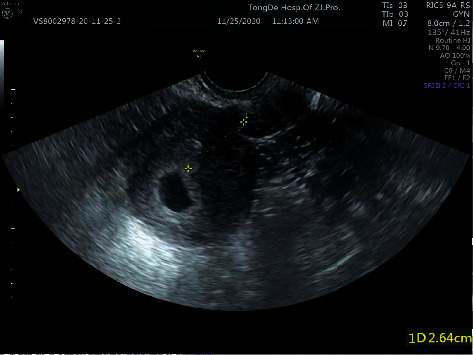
B ultrasound acoustic image of same patient in Figure 1 showing normal intrauterine pregnancy after treatment. The gestational sac is located in the uterus (size: 1.9 × 1.4 × 1.5 cm). The germ and the pulse of the primitive heart tube can be observed.
